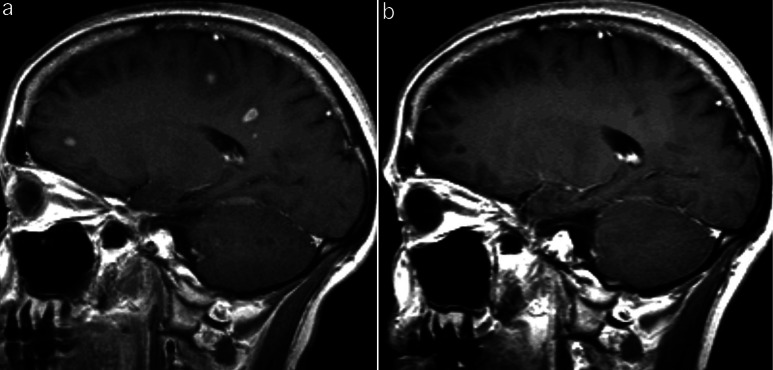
Multiple lesions suspected of multifocal tumor recurrence before (a) and after (b) chemotherapy. T1-weighted post-contrast images.
